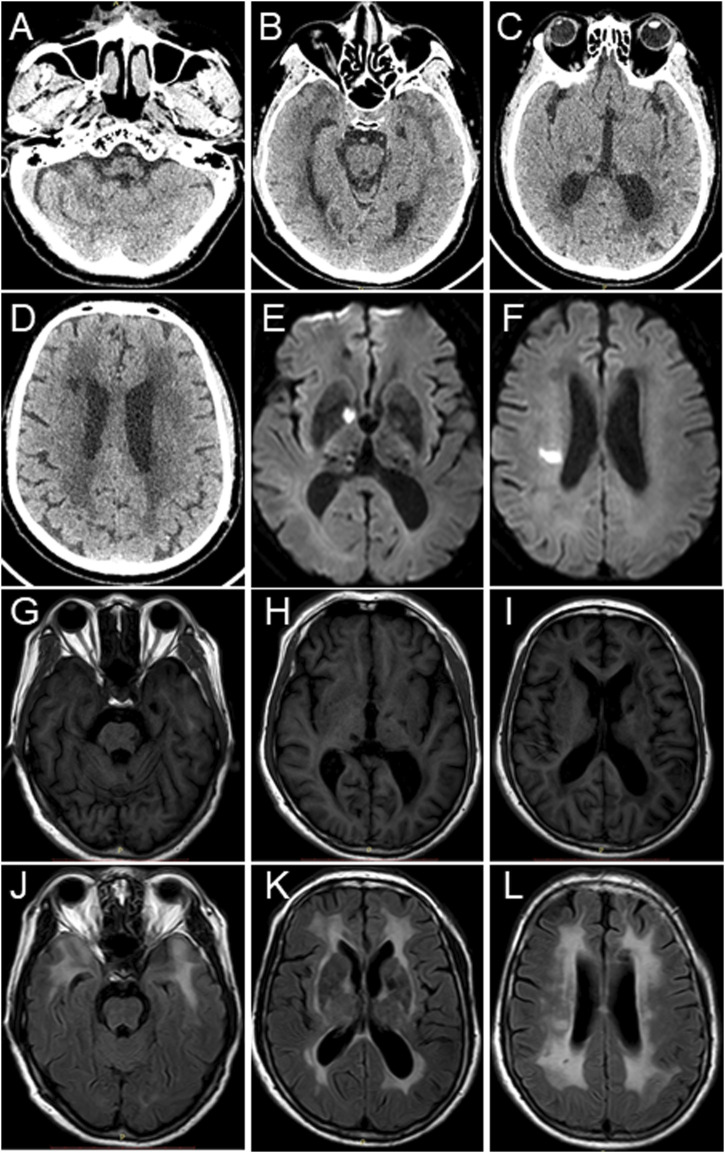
Brain CT scan and MRI images of the proband. (A–D) Brain CT scan suggests multiple lacunar cerebral infarctions, white matter degeneration, and brain atrophy. (E,F) Brain MRI-DWI shows significant hyperintensity signals. (G–I) Decreased signal intensities were observed in the brain stem, basal ganglia, and thalamus in the T1-weighted MRI images. (J–L) T2-weighted and fluid-attenuating inversion recovery (FLAIR) brain MRI revealed symmetrical white matter lesions in the temporal pole, outer capsule, lateral ventricle, and deep brain.
